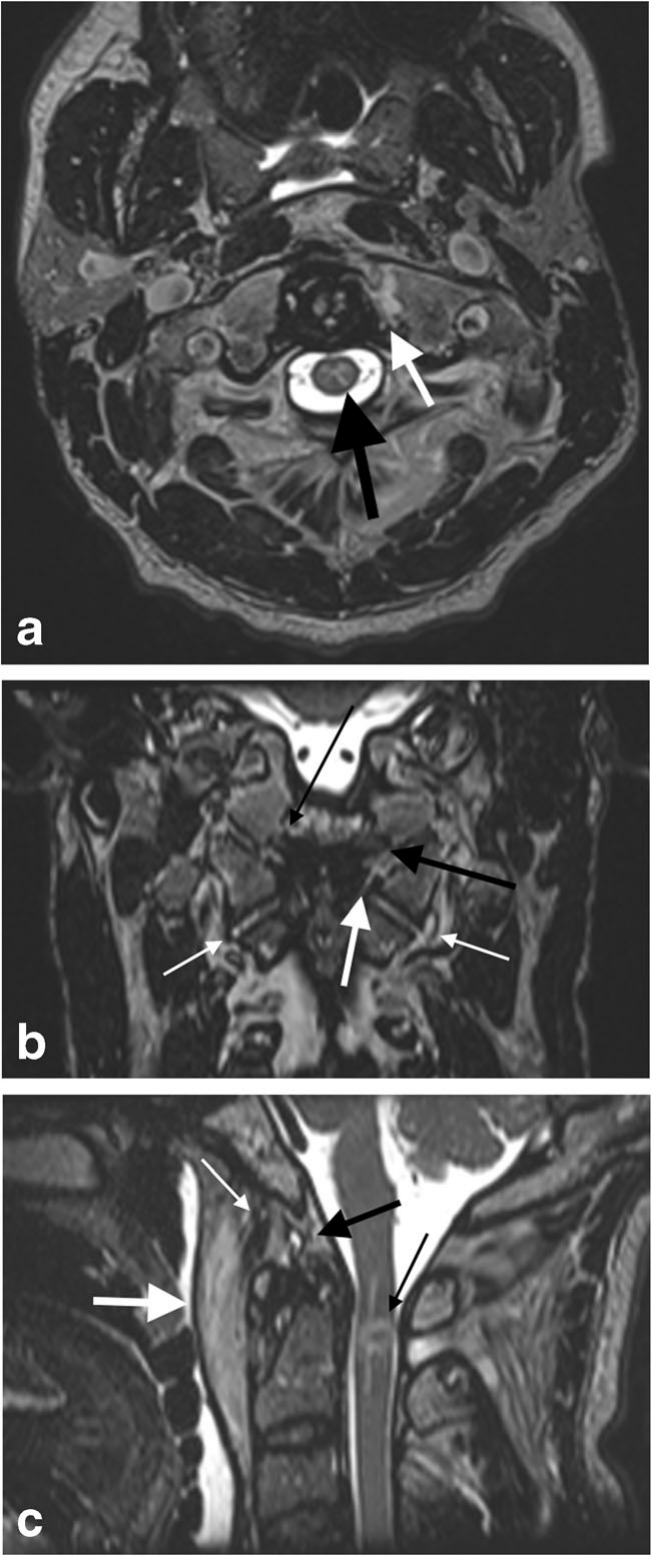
Transverse ligament and alar ligament rupture in a middle-aged male driver of a car involved in a collision with a bus; the victim was quadriparetic and respiratory-compromised at the scene: a axial image of 3D T2 SPACE MRI sequence demonstrates marrow oedema and fracture of the left anterior arch of the atlas extending to and involving the medial tubercle attachment of the transverse ligament on this side associated with rupture and detachment of the the transverse ligament at this site (*arrow*). Cord oedema is evident (*black arrow*); b coronal image of the same MRI study demonstrates traumatic signal abnormality and tear of the left alar ligament (*thick black arrow*) as well as the loss of integrity of the left side of the transverse ligament (*thick white arrow*). Some intrasubstance high-signal strain injury is noted affecting the right alar ligament at its condylar attachment (*thin black arrow*). Traumatic effusions are present in the atlanto-occipital and atlanto-axial joints bilaterally but are more marked on the left indication associated with capsular ligament strain injury (*thin white arrows*); c sagittal image of the same MRI study demonstrates haemorrhagic cord injury at the C1-C2 level (*thin black arrow*), abnormal haemorrhagic signal and fullness in the supra-odontoid space (“apical cave”) related to the apical ligament (*thick black arrow*), an intact tectorial membrane but abnormal traumatic signal change in the anterior atlanto-occipital membrane (*thin white arrow*) associated with a large haematoma in the prevertebral space (*thick white arrow*)
